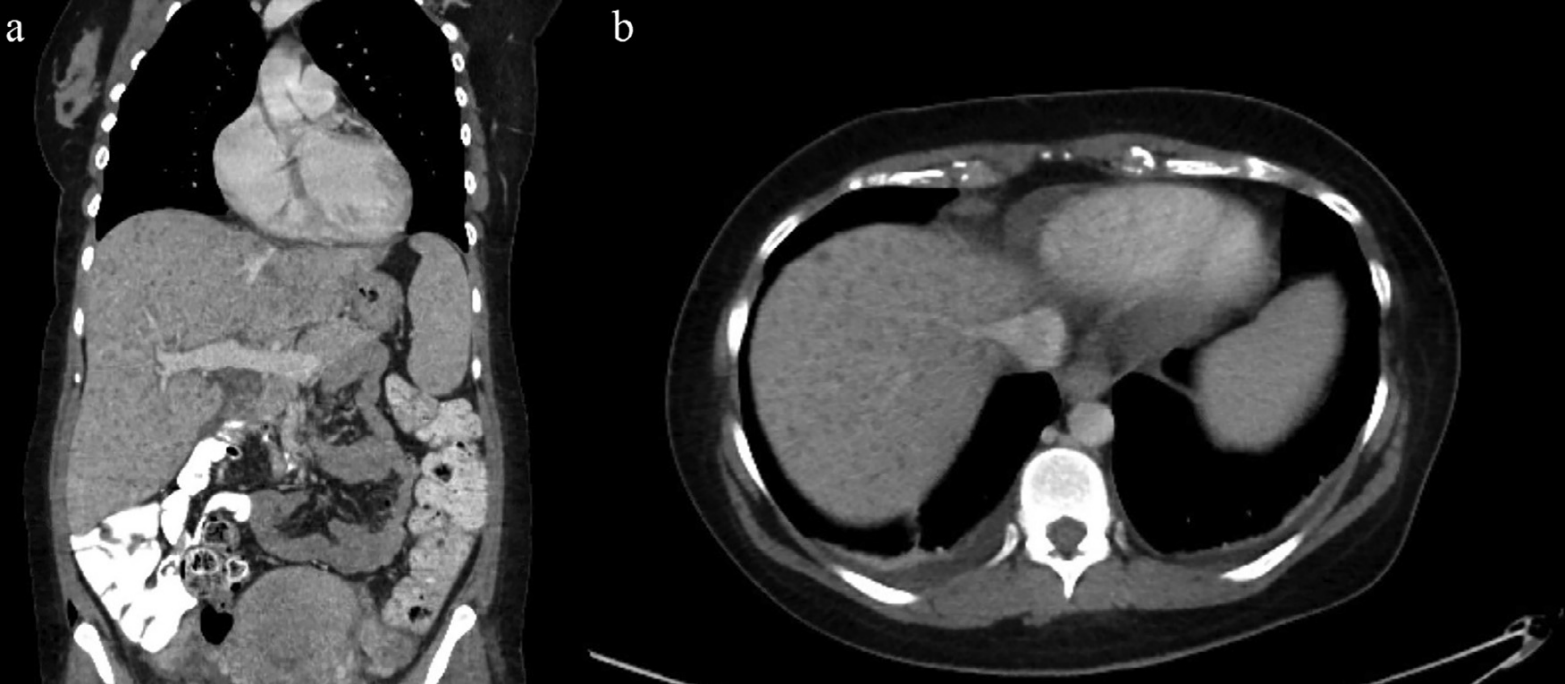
Computed Tomography Angiography (CTA) abdomen with low-density liver lesions, 3a (Coronal view) and 3b (Axial view). Color version of figure is available online.
